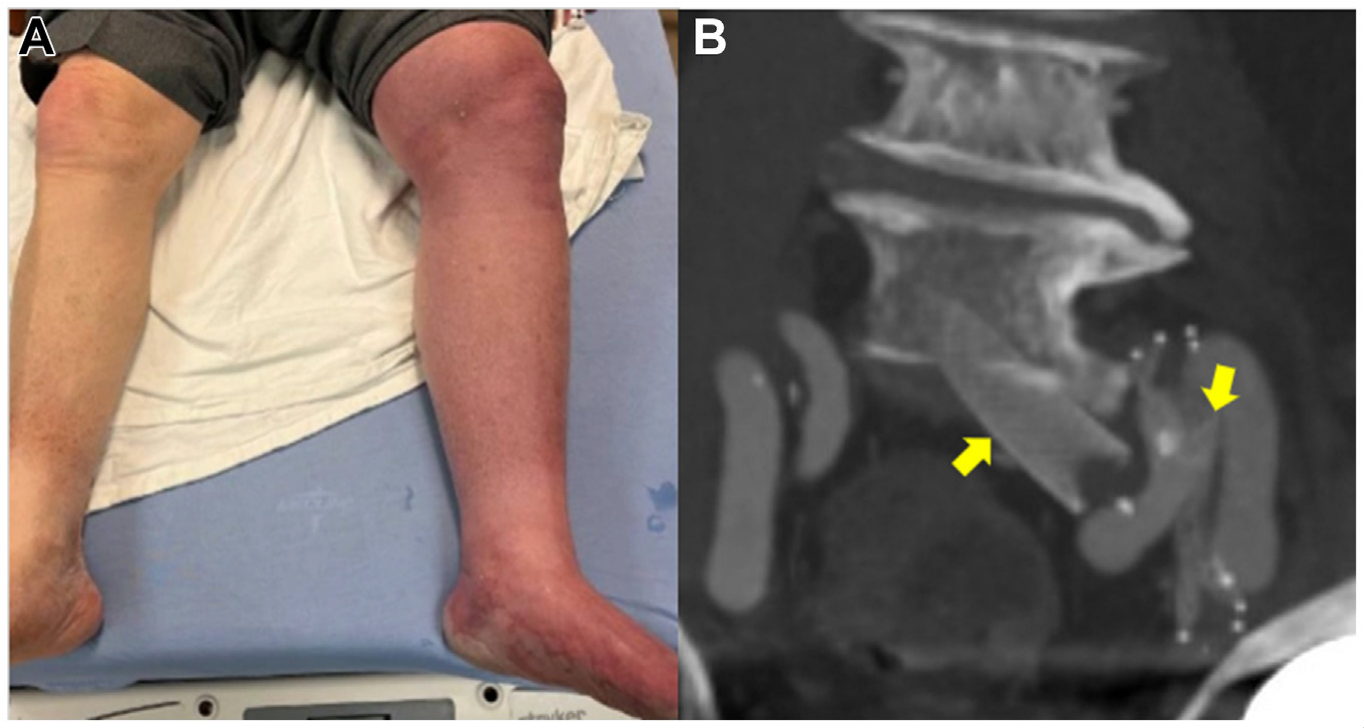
(A) Patient presentation with left leg phlegmasia cerulea dolens. (B) Computed tomography (CT) imaging revealed the two previous iliac stents in discontinuity.
